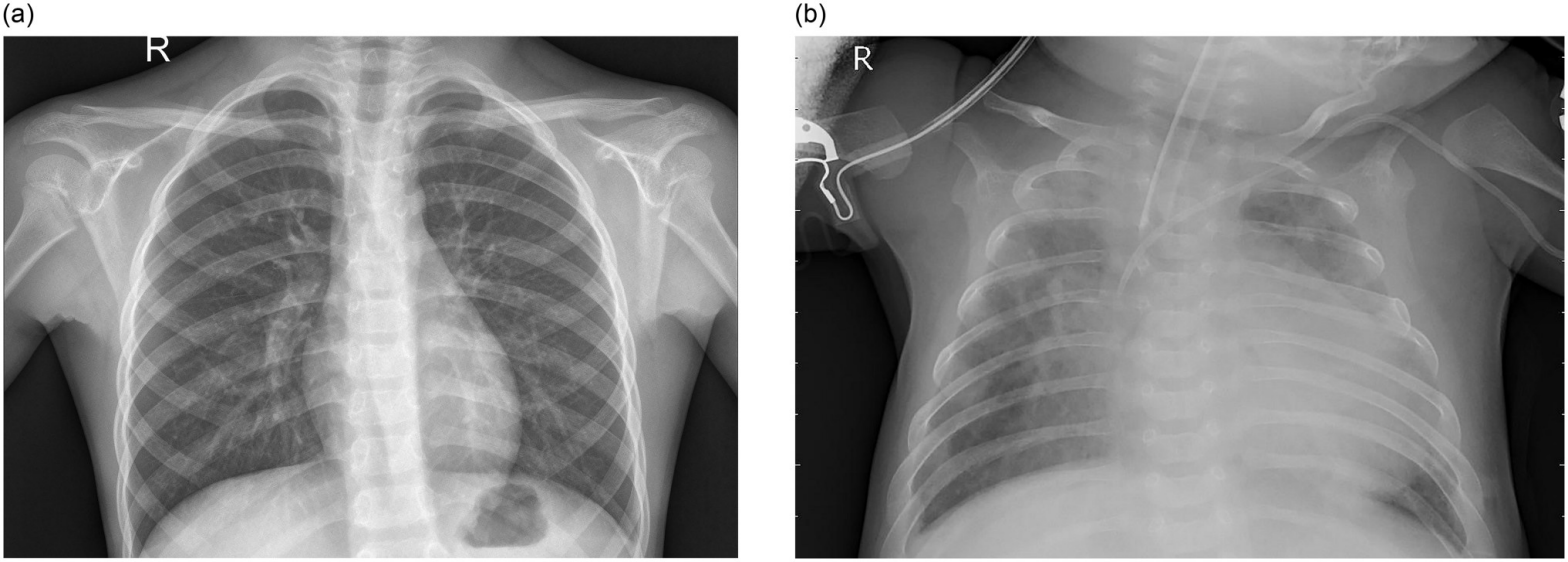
(a): Normal chest X-ray image, (b): Chest X-ray image of patients with pneumonia.
